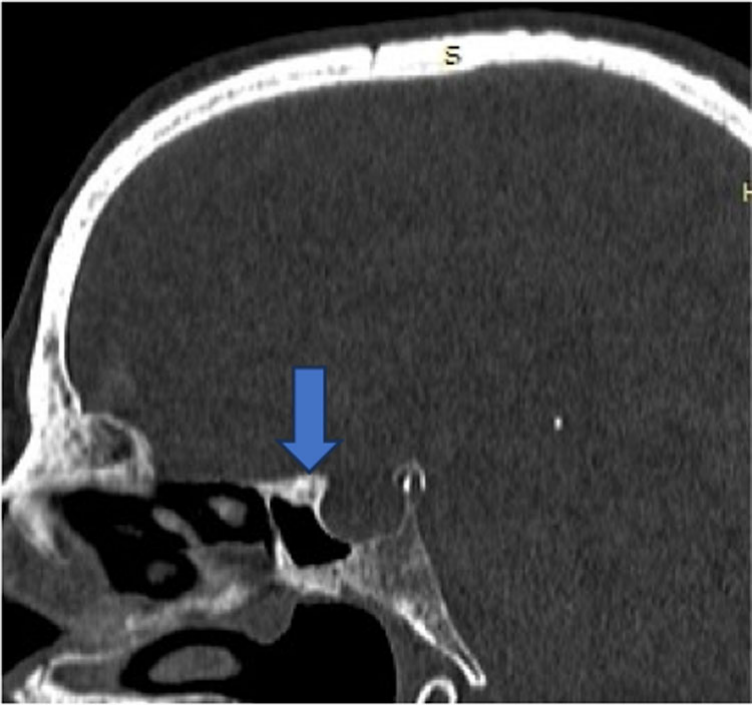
CT Sagittal image shows sellar pneumatization of sphenoid sinus.
